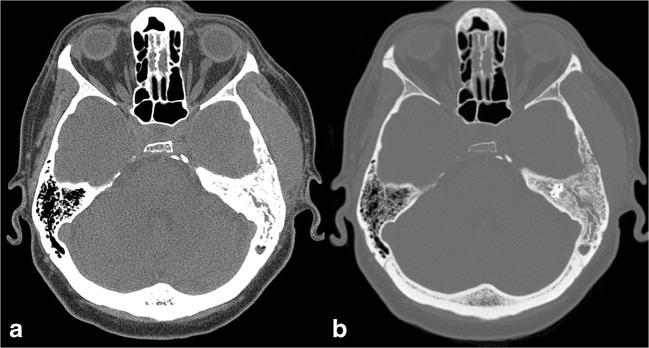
Primary lymphoma of the bone. Axial CT images with soft tissues (a) and bone (b) windows highlight the presence of a bone lesion of the squamous portion of the left temporal bone, with lytic permeative pattern, extending to temporal extracranial soft tissues
